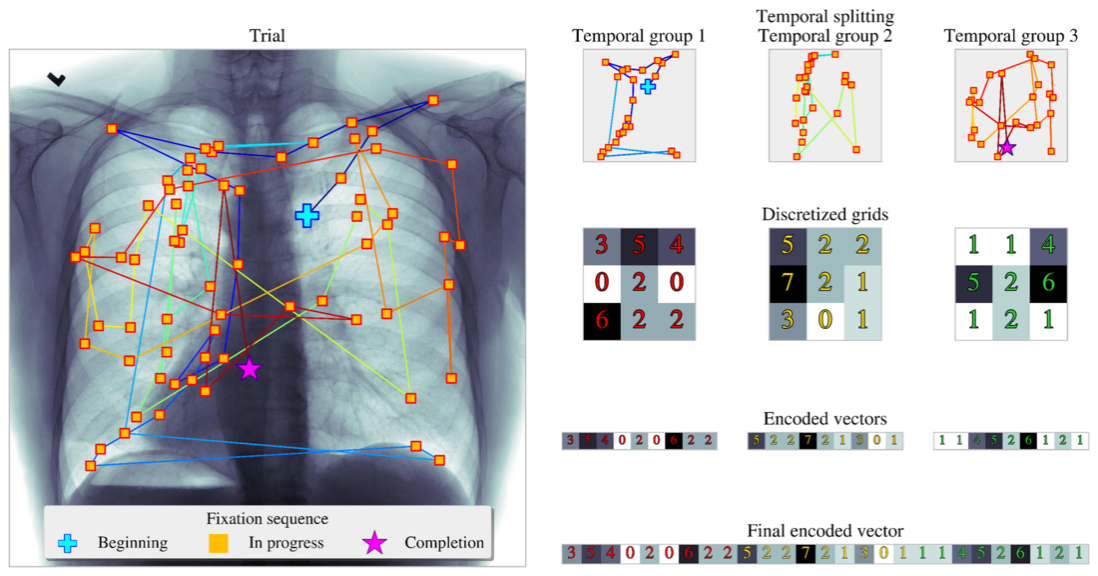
Proposed discretized vector encoding for fixation data. Bins 1, 2, and 3 capture fixations in a preserved spatial dimension across different temporal windows. Each row represents a temporal bin, and within each bin, the chest x-ray image is divided into spatial grids. The fixations are counted within each grid cell, providing a detailed representation of the radiologist’s visual search pattern over time.
